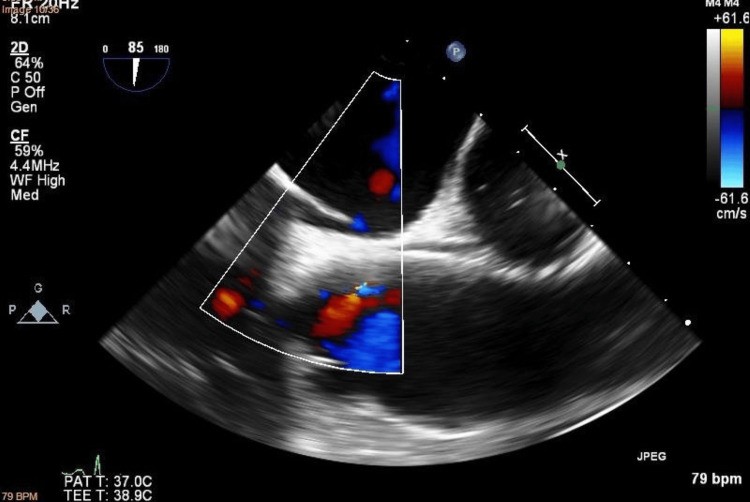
No signs of patent foramen ovale on transesophageal echocardiography with color Doppler
